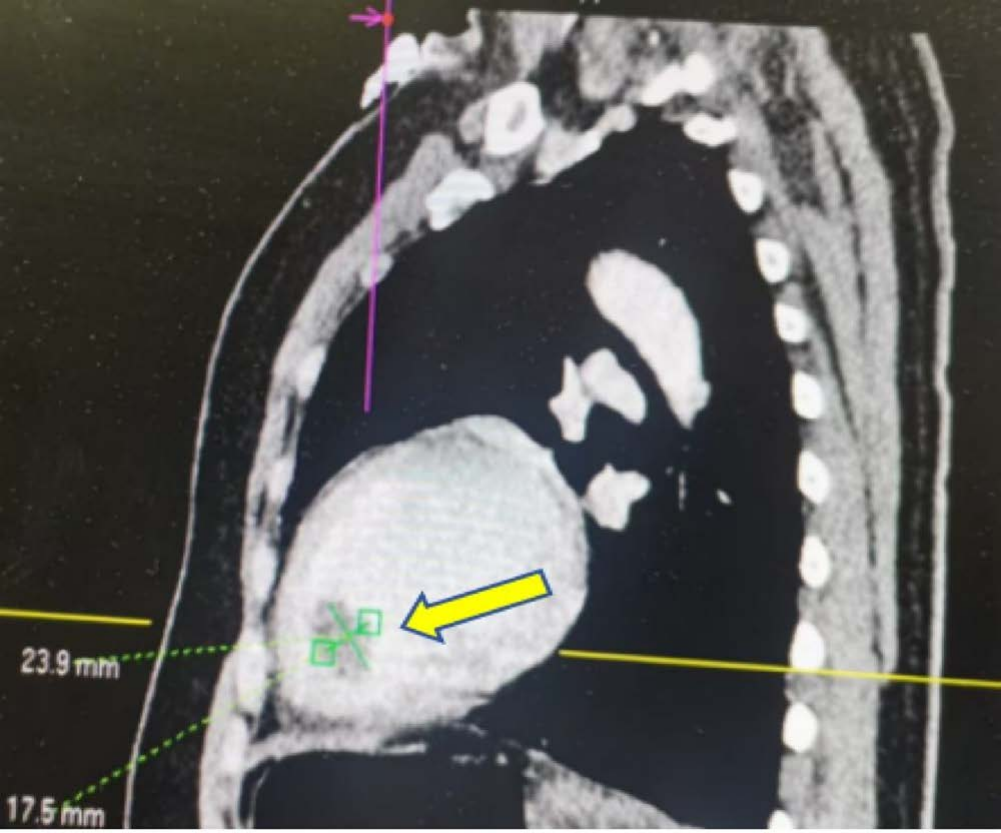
Contrast-enhanced CT scan showing an LV thrombus (yellow arrow). CT = computed tomography, LV = left ventricle.
